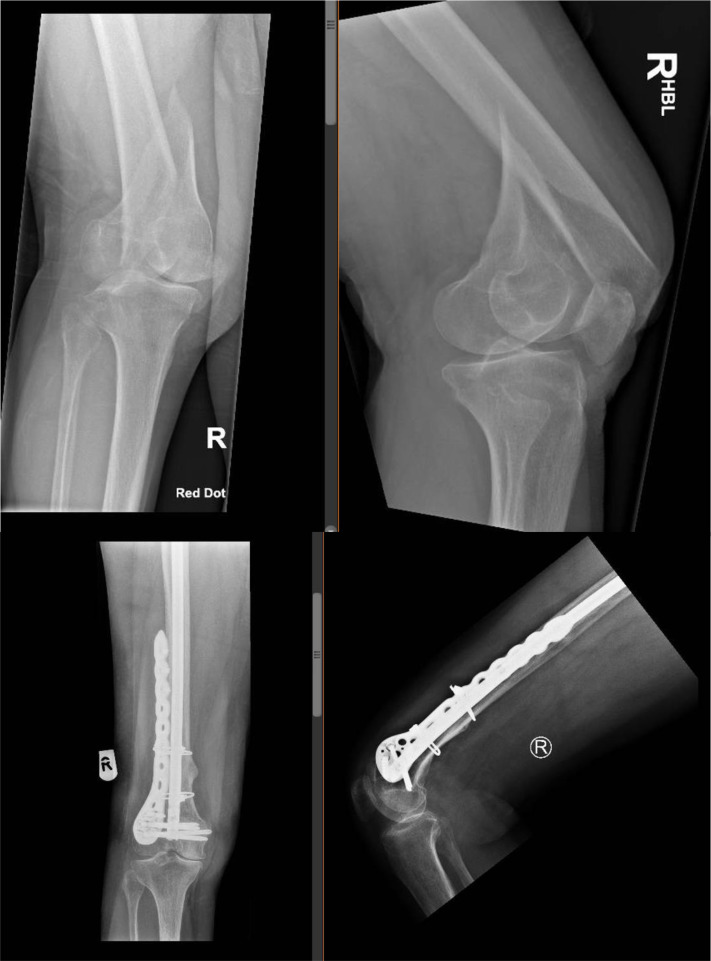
Pre-operative and post-operative radiographs of distal femur fracture (native joint) treated with linked nail/plate construct.
